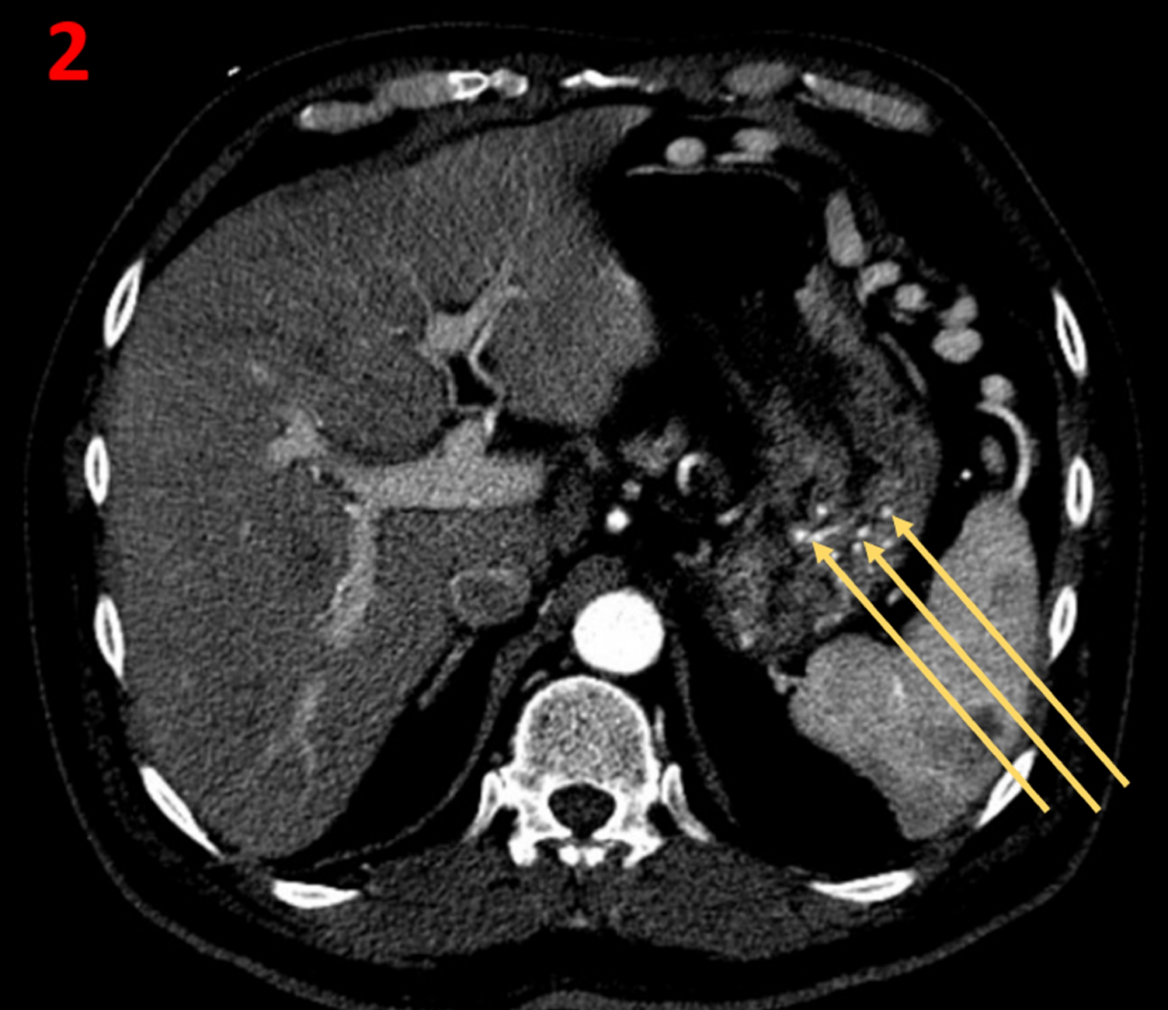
Gastric fundal wall as seen on axial abdominal CT CT: computed tomography Contrast-enhanced axial CT in the arterial phase demonstrates multiple large arteries (yellow arrows) in the gastric fundal wall with some vessels coursing in close approximation to the submucosa
